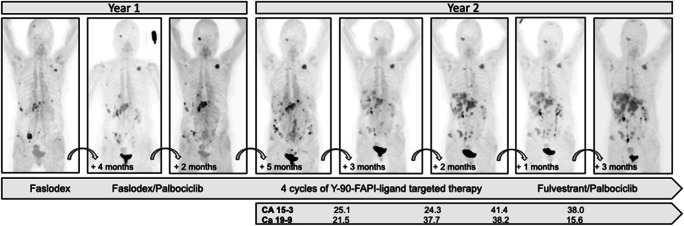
A 79-year-old female with metastasized breast and colon cancer underwent within 1.5 years eight ^68^Ga-FAPI-PET/CT with three different derivates (FAPI-02, FAPI-04, FAPI-46). During that time interval, mainly palbociclib and four cycles of Y-90-FAPI-radioligand therapy were applied. The progression markers CA 15-3 and CA 19-9 were monitored showing a temporary stable and even regredient disease, which is in concordance with FAPI uptake presented in the PET/CT. However, after a while, the patient possibly developed a resistance against palbociclib or radioligand therapy, presenting with possible progressive disease leading to a change of therapy
